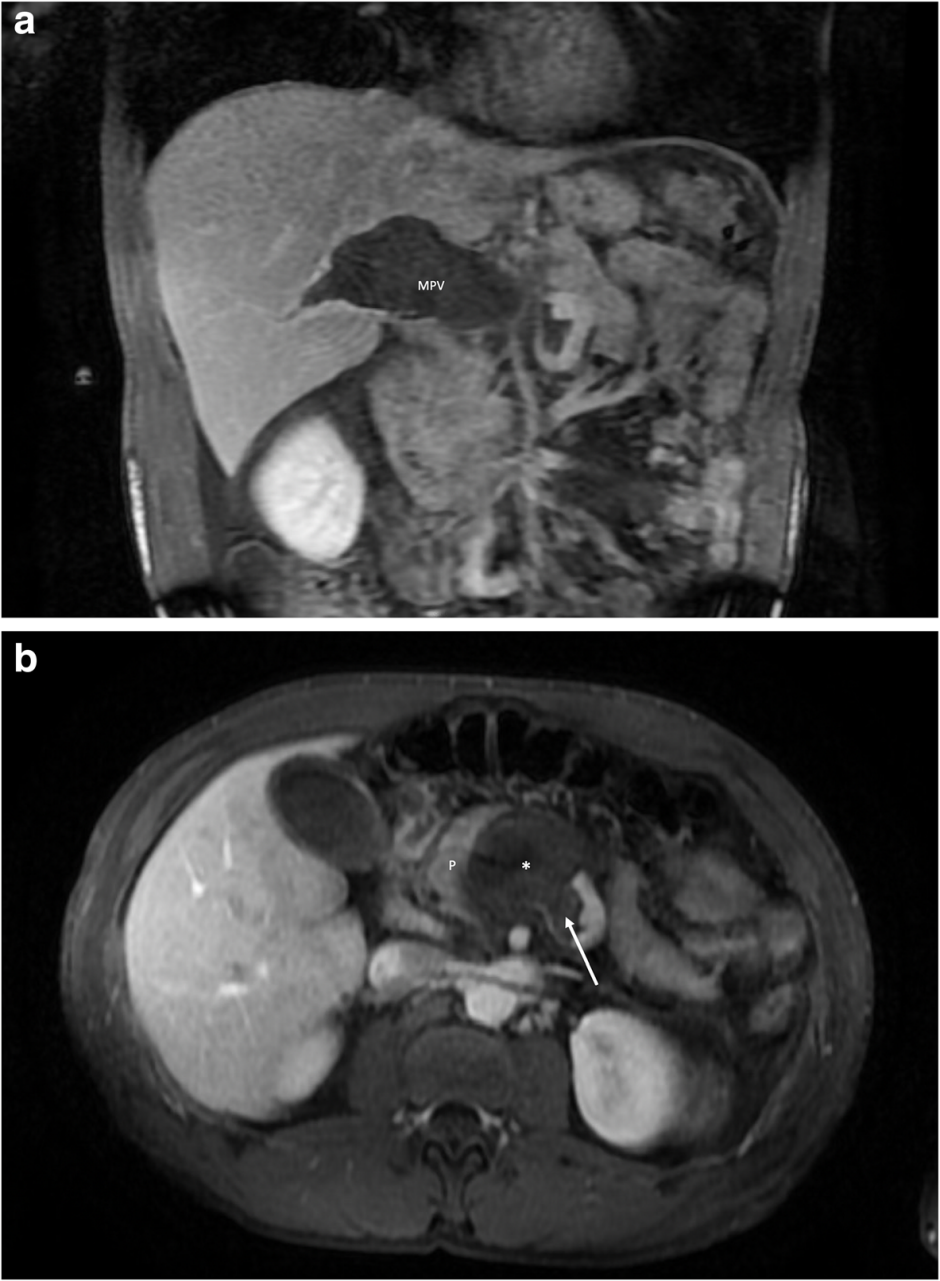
A Coronal contrast-enhanced T1 fat-saturated MRI image demonstrates the thrombosed extrahepatic portal venous component (MPV) of the large portomesenteric aneurysm. Thrombus also extends into the right portal vein. B Axial contrast-enhanced T1 fat-saturated MRI image demonstrates occlusive thrombus at the aneurysmal portosplenic confluence (*) abutting the adjacent pancreas (P). Non-occlusive thrombus is present in the central aspect of the splenic vein (arrow)
